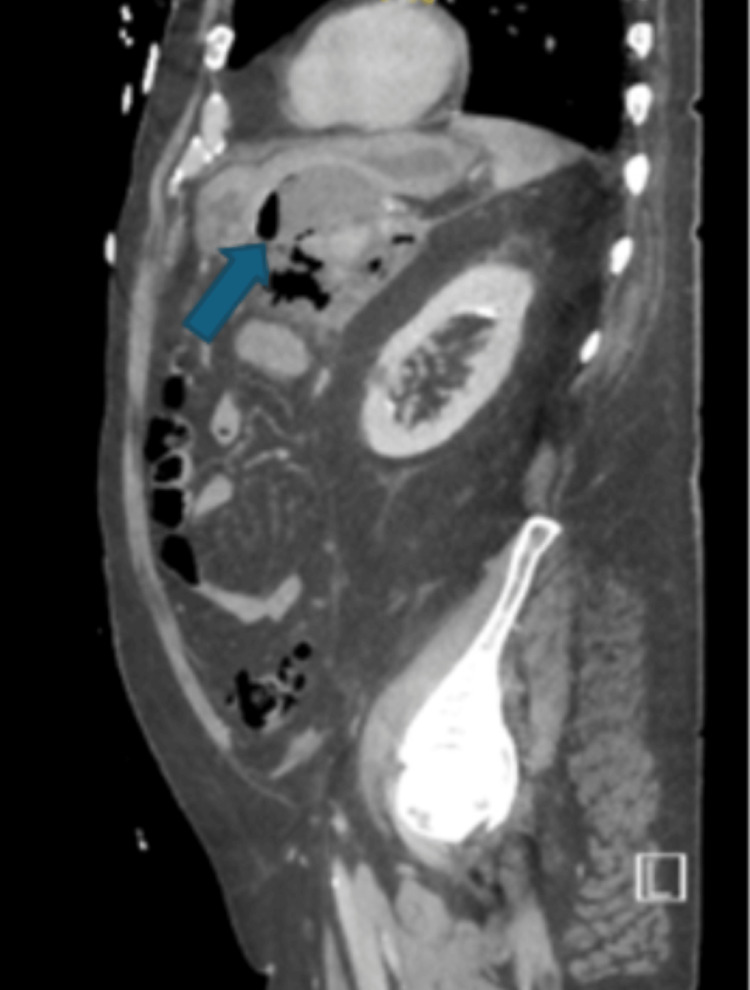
CT angiography of the abdomen and pelvis with and without contrast (sagittal view) showing pancreatic necrosis: peripancreatic gas-containing fluid collection measuring up to 18.5 cm with the fluid collection abutting the greater curvature of the stomach and proximal small bowel loops CT: computed tomography
